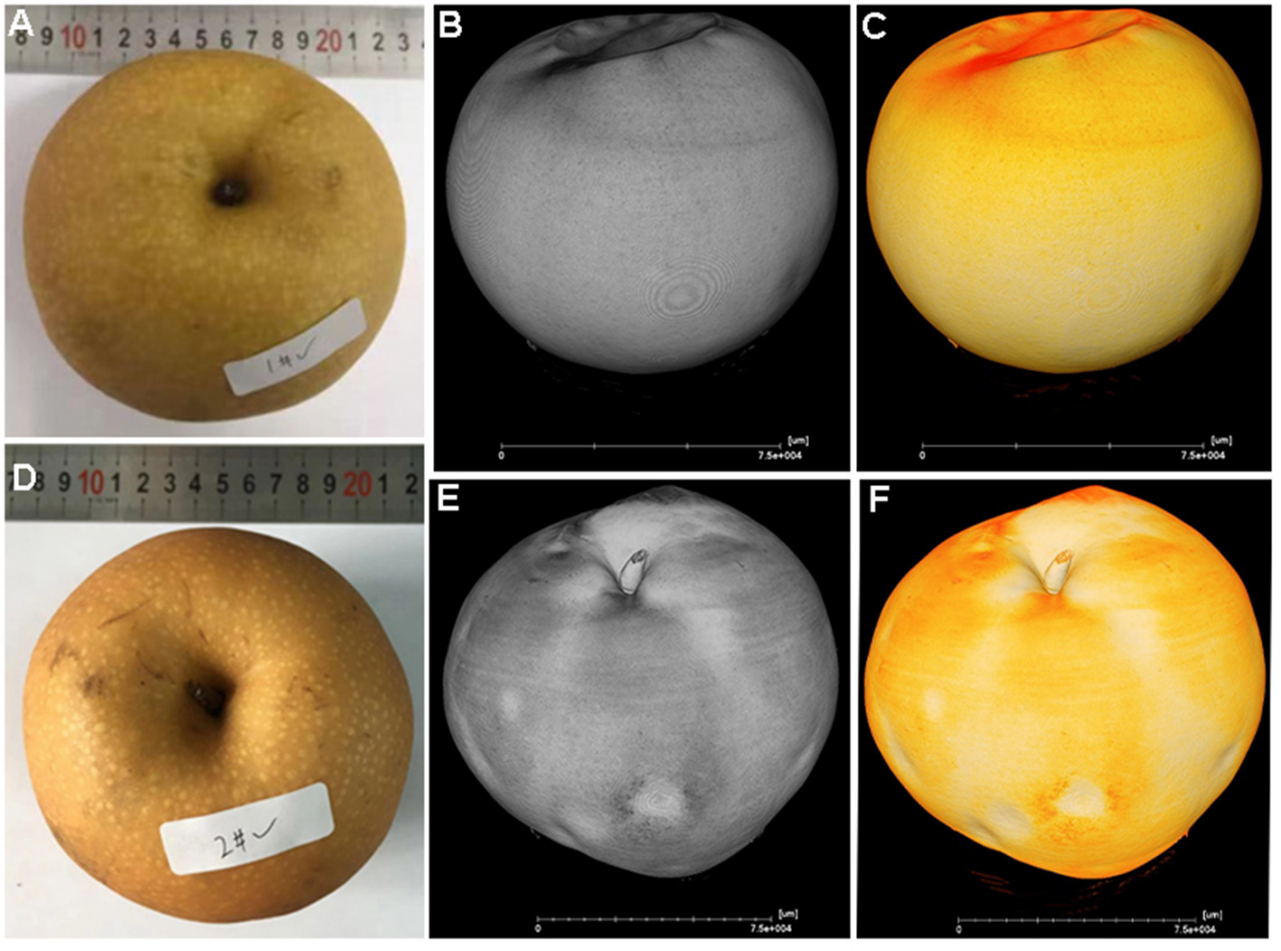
Comparison of the appearance of healthy and cork spotted “Akizuki” fruit at harvesttime. (A) Healthy fruit. (B,C) Gray and yellow rendering of healthy fruit with X-ray scanning, respectively. (D) Cork spotted fruit. (E,F) Gray and yellow rendering of cork spotted fruit with X-ray scanning, respectively.
